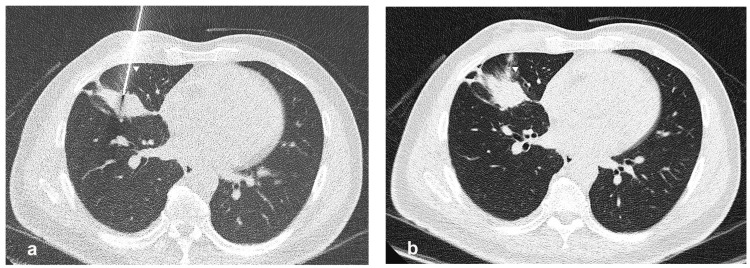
(a) Axial CT scan shows the pulmonary lesion at the right middle lobe with the 17-gauge coaxial biopsy needle (arrowhead) in the lesion. (b) Post-biopsy CT scan performed after track embolization with gelatin sponge torpedo showed a radiolucent track along the needle path (arrowhead).
